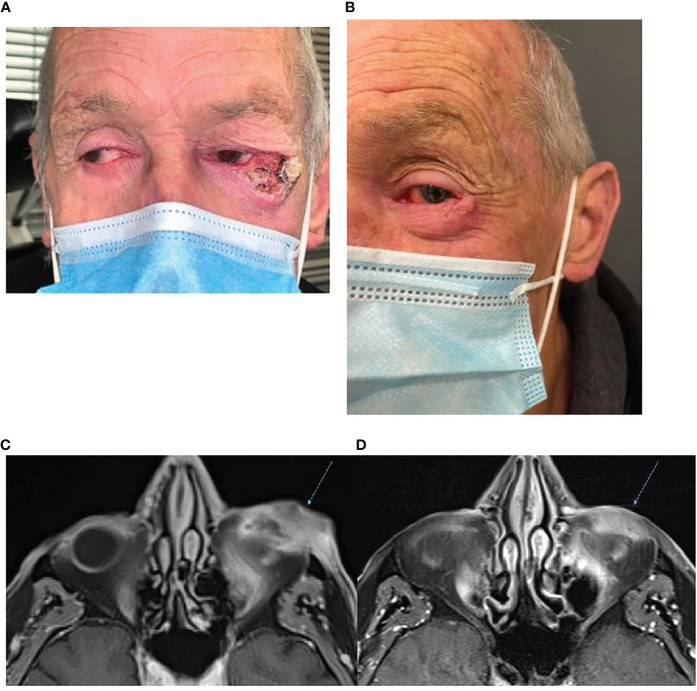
(A) Baseline photography of left lateral canthus CSCC prior to commencement of immunotherapy. (B) Photography of the lesion post two cycles of cemiplimab 350 mg/3-weekly demonstrating an excellent clinical response to therapy. (C) Baseline, left, MRI post-contrast T1 Fat Sat demonstrating 38 × 18-mm inferolateral canthus enhancing mass. (D) MRI post contrast T1 Fat Sat, post three cycles of cemiplimab 350 mg/3-weekly demonstrating reduced size and enhancement.
